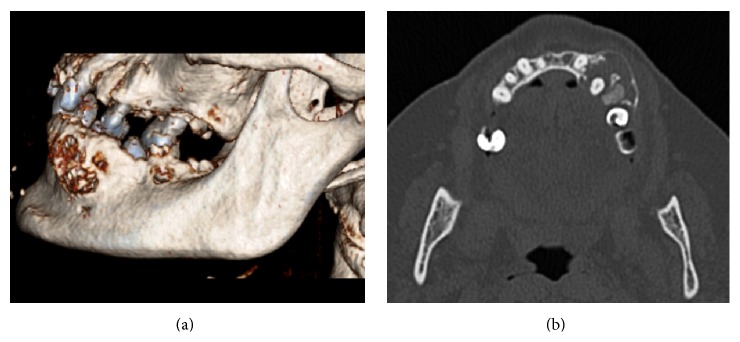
(a) Three-dimensional computed tomography (CT) reconstruction showing an expansion of the left-side of the mandible with some erosion areas on buccal cortical surface. (b) Axial CT image showing a low-density multilocular lesion.
